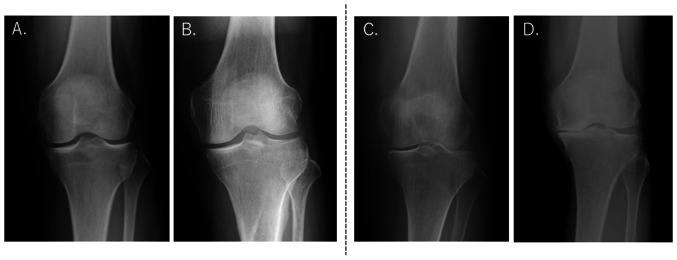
Representative X-ray images of knees from subjects who were (A) KL grade 0, (B) KL grade 1, (C) KL grade 2 and (D) KL grade 4. Selected for analysis were KL grades 0-1 and excluded from analysis were KL grades ≥2. KL, Kellgren-Lawrence classification.
